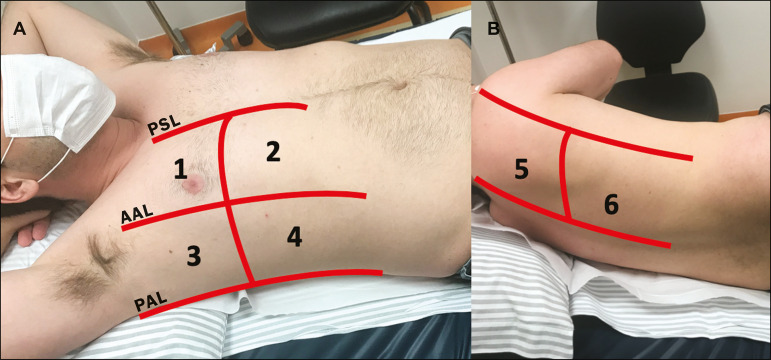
Patient positioning for the examination, anatomical references, and evaluated regions. A: Horizontal supine position. B: Left lateral position. PSL, parasternal line; AAL, anterior axillary line; PAL, posterior axillary line. 1, anterosuperior region; 2, anteroinferior region; 3, upper lateral region; 4, lower lateral region; 5, posterosuperior region; 6, posteroinferior region.
